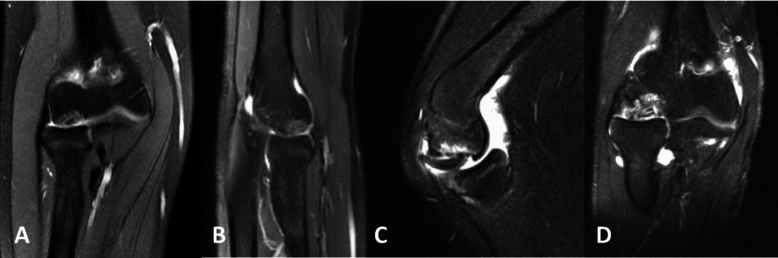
MRI examples of unstable osteochondritis dissecans lesions of the capitellum. A Coronal image of a small (< 1 cm^2^), centralized lesion. B Sagittal image of a small (< 1 cm^2^), centralized lesion. C Sagittal image of a large (> 1 cm^2^) lesion. D Coronal image of a large (> 1 cm^2^) lesion
